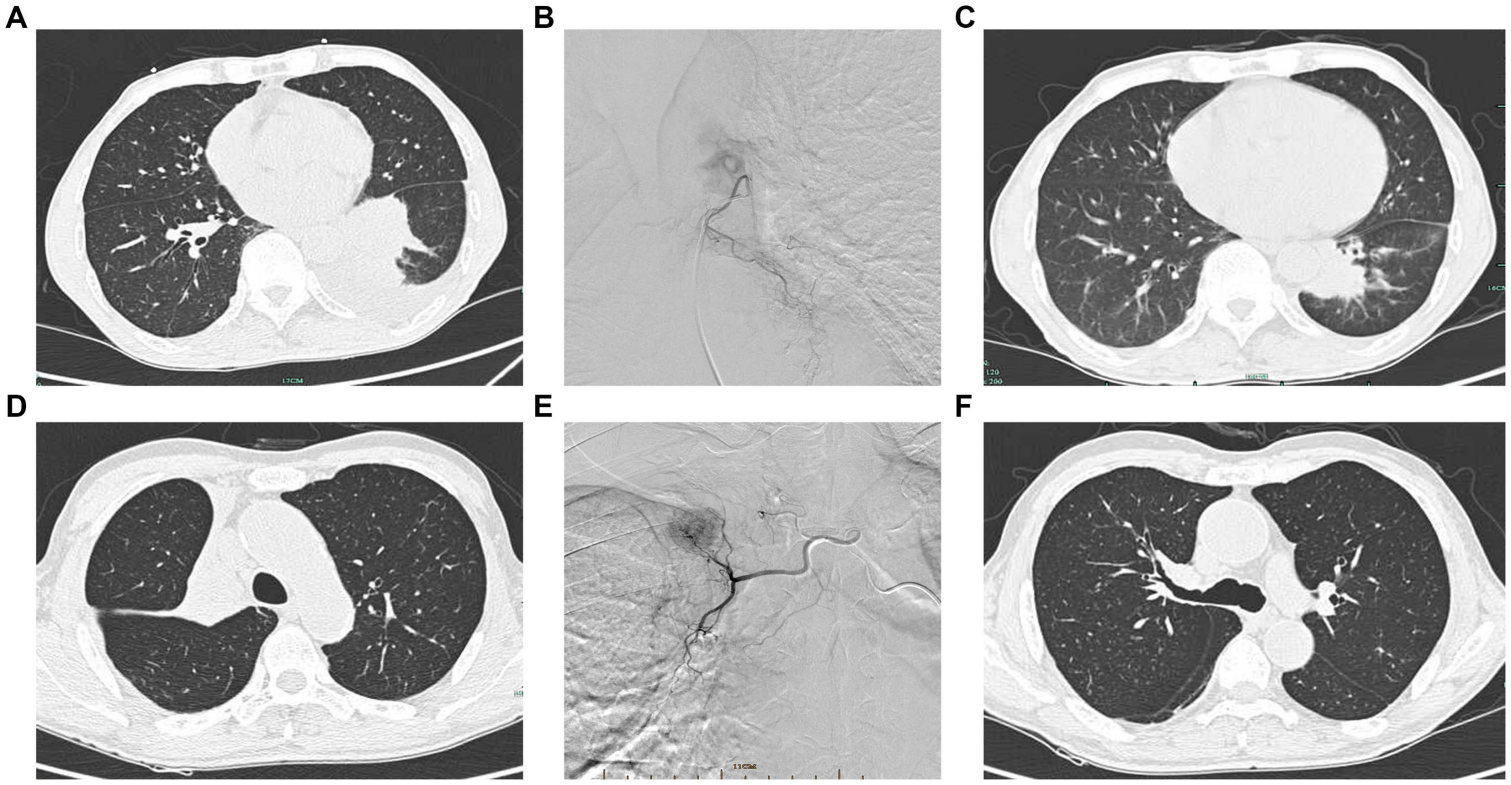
Interventional procedure images and computed tomography images before and after intervention. (A) Pre-interventional image of a case of squamous carcinoma of the left lower lung. (B) DSA showing left bronchial artery supplying left lower lung cancer. (C) Significant shrinkage of the left lower lung mass after intervention. (D) Squamous carcinoma of the right upper lobe opening with right upper lung atelectasis. (E) DSA showing right bronchial artery supplying the right upper lung mass. (F) Regression of the tumor of the right upper lobe opening and lung reopening after intervention.
